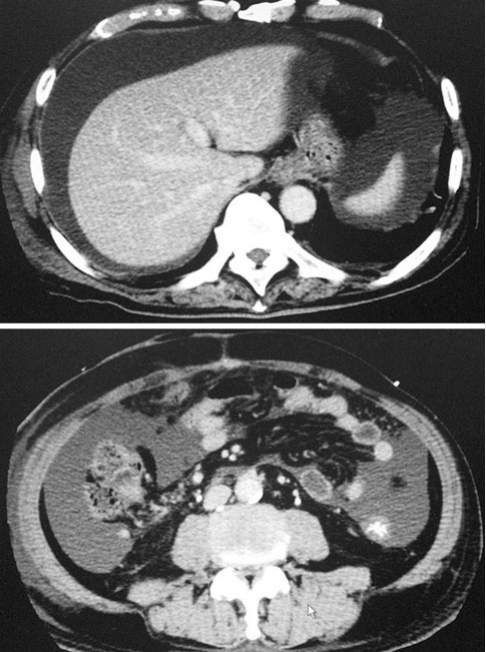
Abdominal computed tomography performed 3 weeks after surgery. Massive ascites was seen in the abdomen
